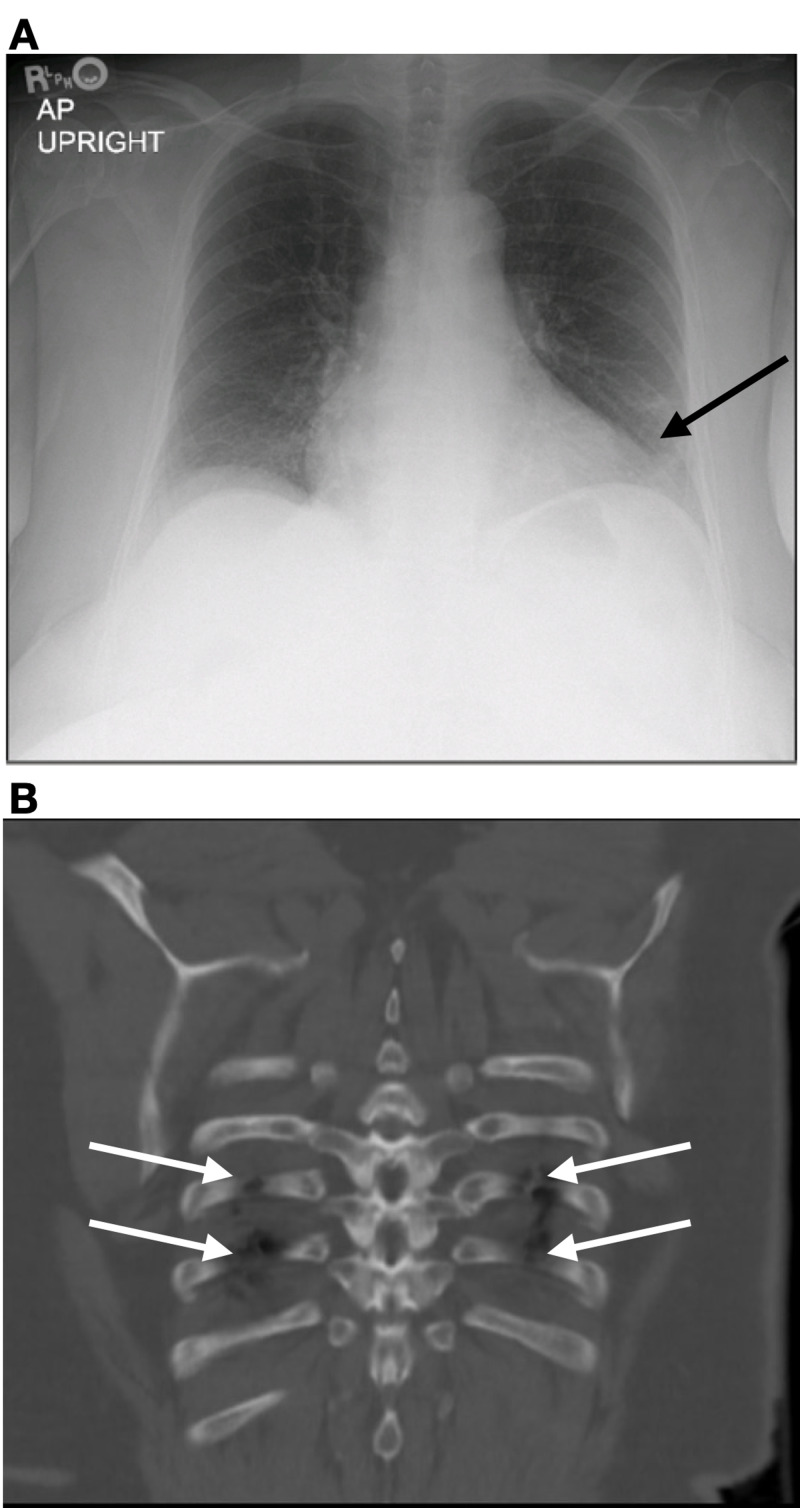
Presenting imaging (A) Anterior-posterior (AP), frontal chest radiograph. Findings: subsegmental atelectasis in the left lung base without acute cardiopulmonary pathology. (B) Computed tomography. Findings: multiple, anterior, rib fractures bilaterally without other organ damage.
